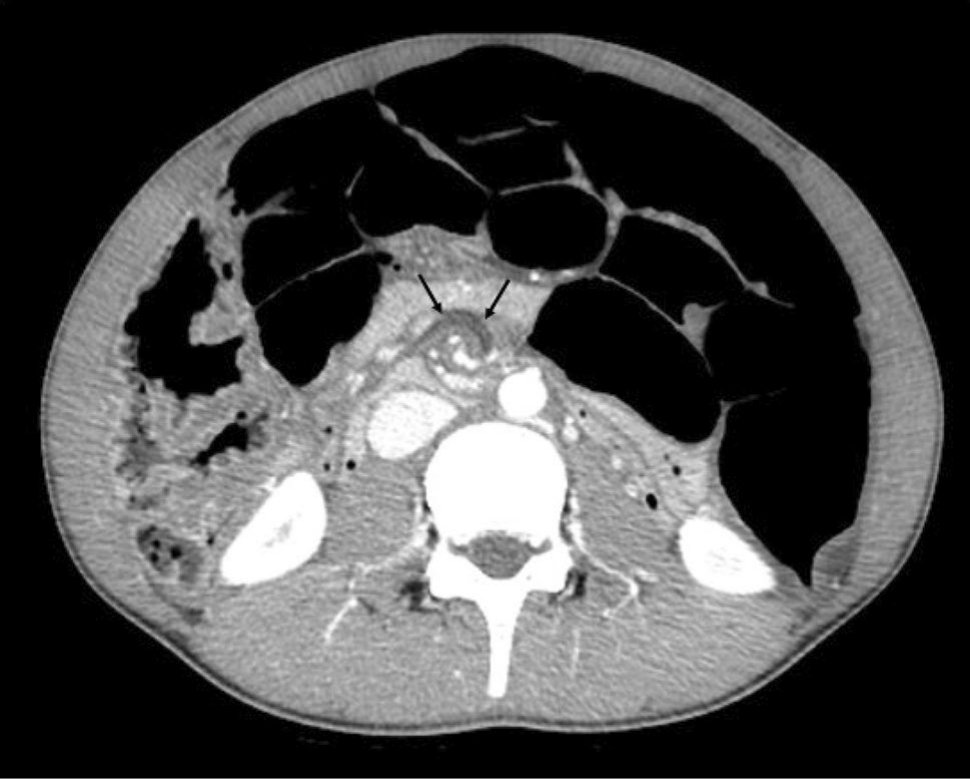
Enhanced computed tomography showing colonic dilation, narrowing of superior mesenteric vein, and whirlpool sign (arrows) of mesentery that suggests small intestinal volvulus
